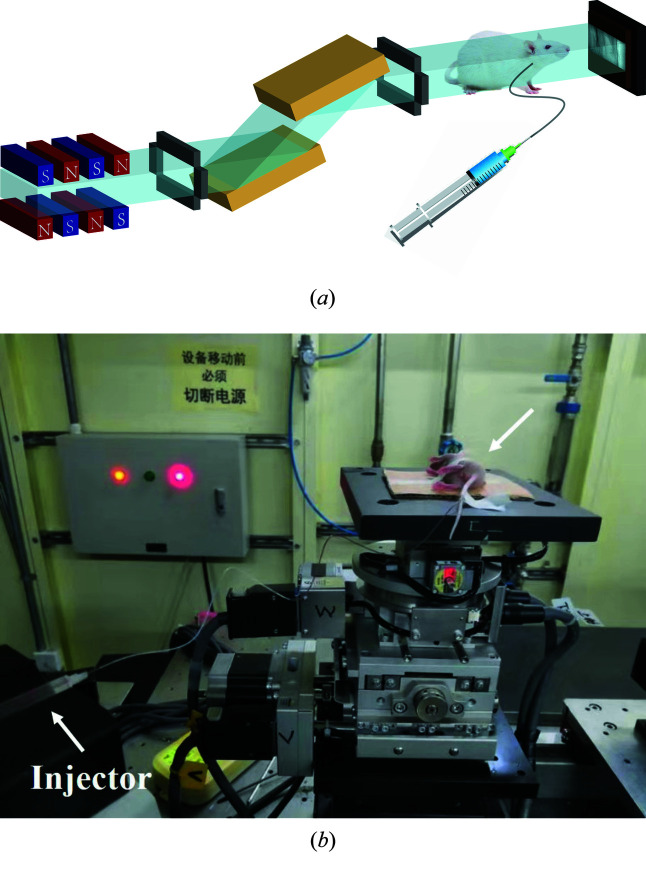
The experimental setup for MCXI with synchrotron X-rays, where (*a*) is the optical layout and (*b*) is a picture of the sample stage.
